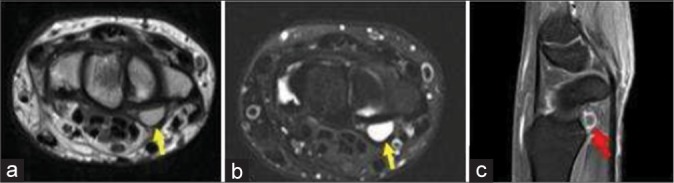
A 35-year-old man with ganglion cyst who presented with painless swelling over the proximal hand. (a) Non-contrast T1-weighted axial image showing a well-defined hyperintense lesion on the volar surface due to proteinaceous contents (yellow arrow). (b) Axial T2-weighted Magnetic resonance image at the level of wrist confirms a well-defined cystic lesion in volar surface adjacent to the flexure retinaculum (yellow arrow). (c) Fat saturated post-contrast sagittal T1 image shows rim enhancement of the cyst (red arrow).
